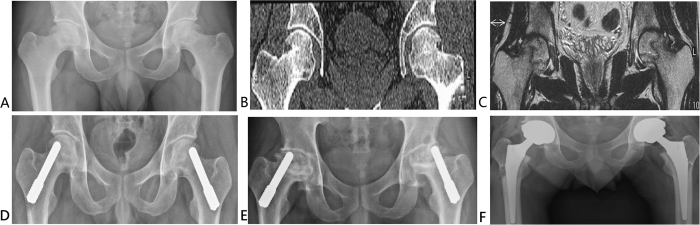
Anteroposterior X-ray and computed tomography examination of a representative case of 29-year-old man with bilateral Association Research Circulation Osseous stage III osteonecrosis of the femoral head (ONFH) (A,B). Preoperative magnetic resonance image (coronal section, T1WI) of the patient showing bilateral necrosis of the bone marrow and the cortical bone. CJFH type is based on the three pillars. The bilateral sides are both type L3. Necrosis involves all three pillars (C). Porous tantalum implants were inserted in the bilateral hips to treat ONFH (D). Anteroposterior radiographic films showing that the bilateral hips progressed collapsed from ARCO stage III to ARCO stage IV 42 months after a porous tantalum implantation surgery (E). Two porous tantalum implants inserted in the bilateral hips required conversion to THA after 42 months (F).
